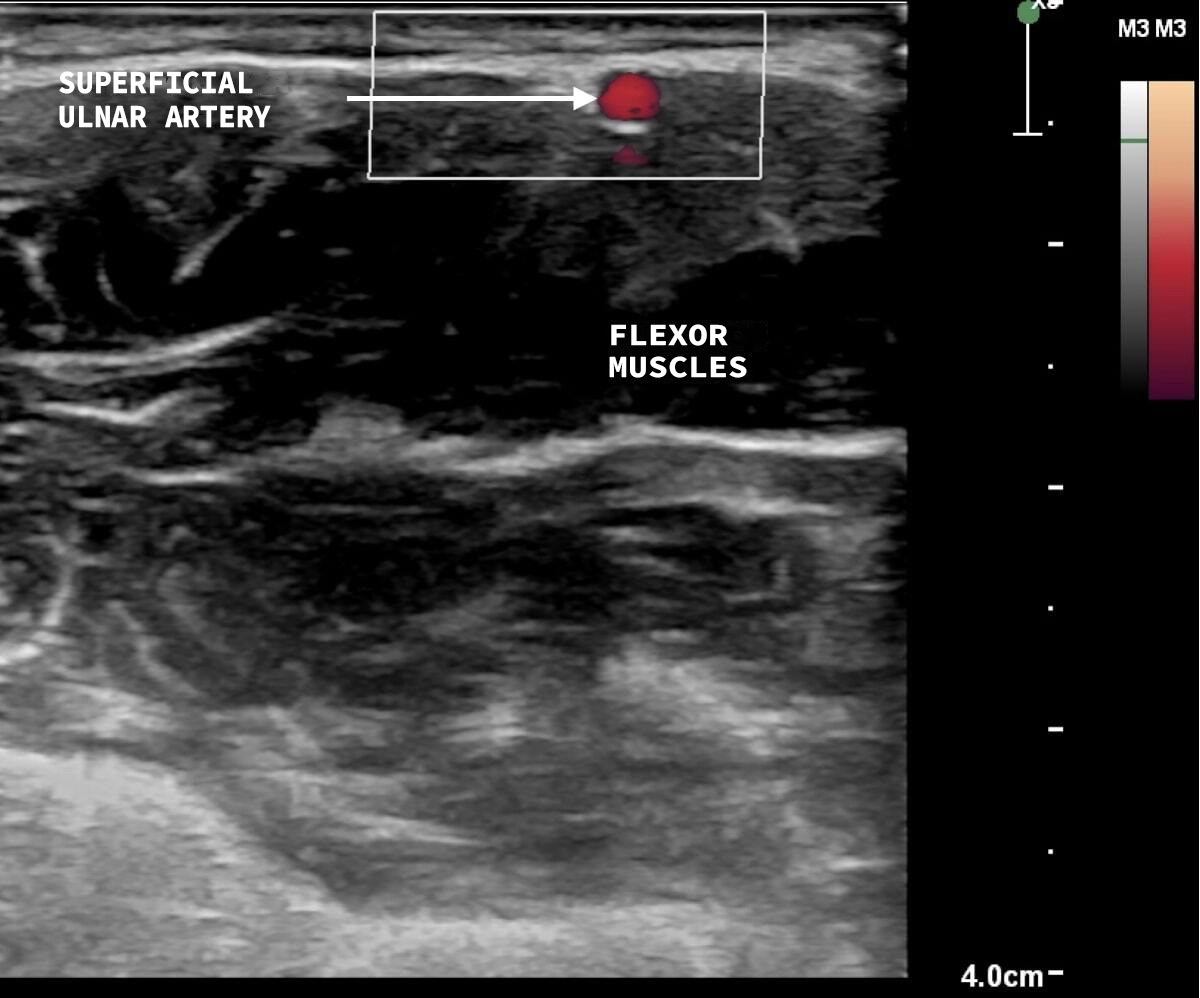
Vascular ultrasound, transverse view, with superficial ulnar artery anatomic variant present, coursing above the flexor muscles of the forearm.
